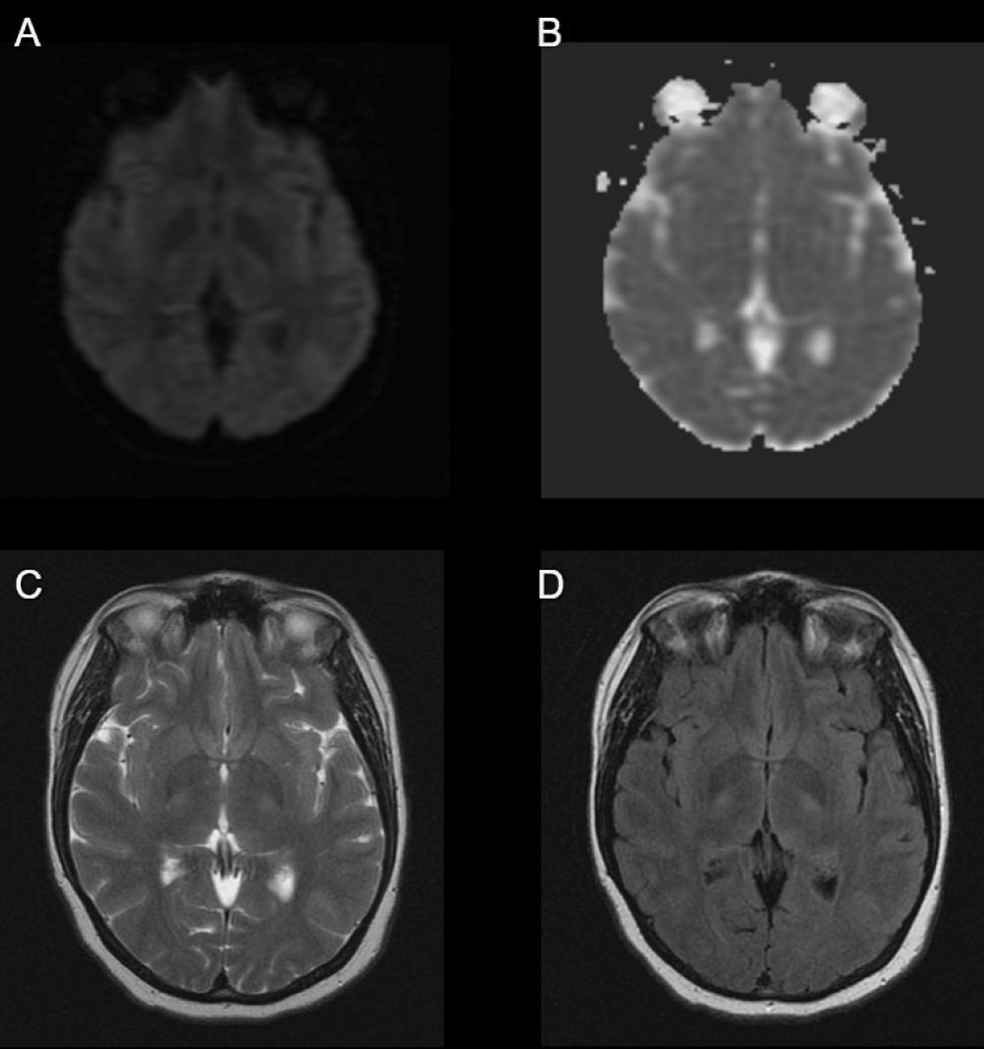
MRI brain without contrast one year after treatment MRI brain without contrast showing complete interval resolution of the abnormal hyperintensities within the bilateral thalami on axial DWI, T2, and FLAIR sequences (A, C, D). There was no abnormal signal on the axial ADC sequence (B). No evidence of diffusion restriction. DWI, diffusion-weighted imaging; FLAIR, fluid-attenuated inversion recovery; ADC, apparent diffusion coefficient.
